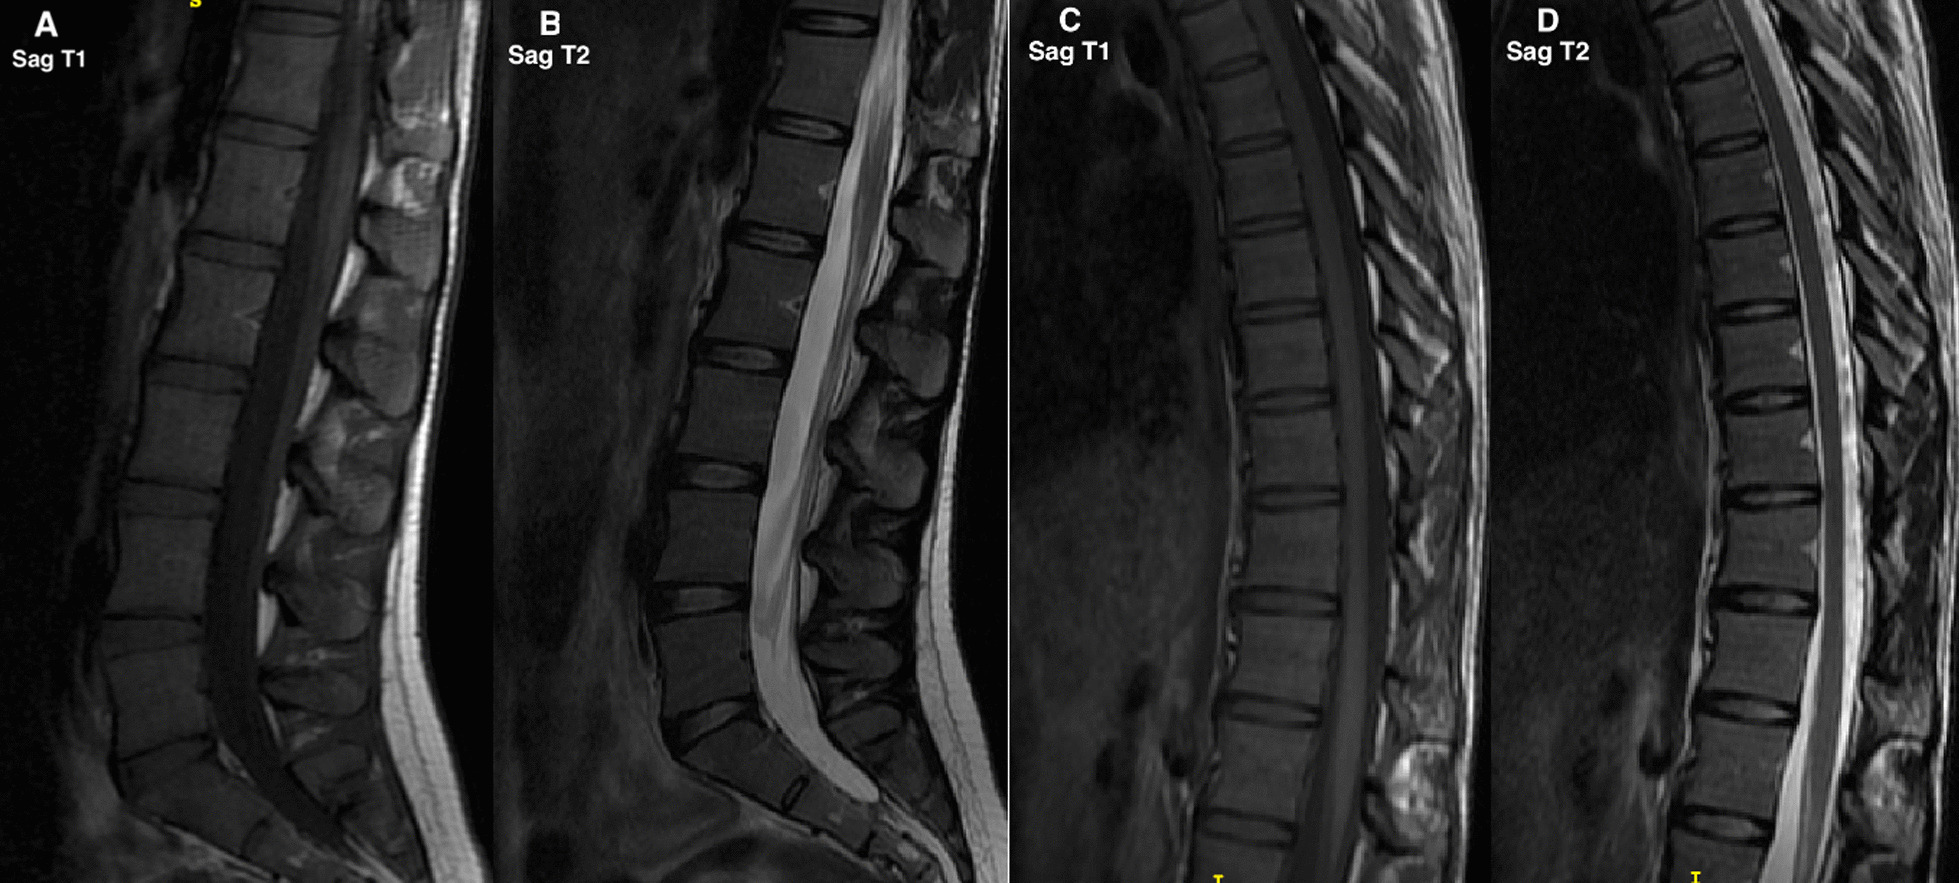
MRI of a female climber in the 20s with few prevalent spinal changes. The lumbar and thoracic spine (A–D) show few signs of spinal changes of the endplates and IVDs
